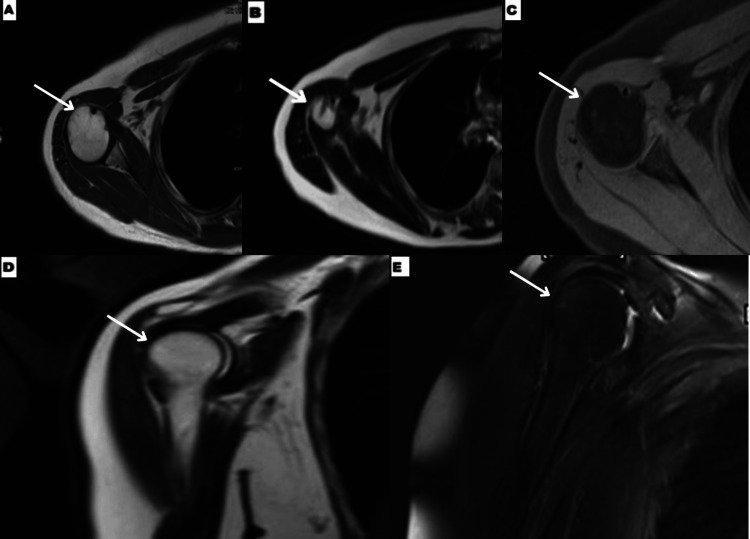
MRI findings - 2 MRI of the humerus on 2/29/24 with axial T1 (A, B), axial T1 fat sat post contrast (C), cor T1 (D), and cor T2 fat sat (E) images show complete resolution of prior intramedullary lesion (A, C, D, E arrows). Axial T1 (B) shows residual biopsy tract in the humeral neck (arrow) MRI: magnetic resonance imaging
